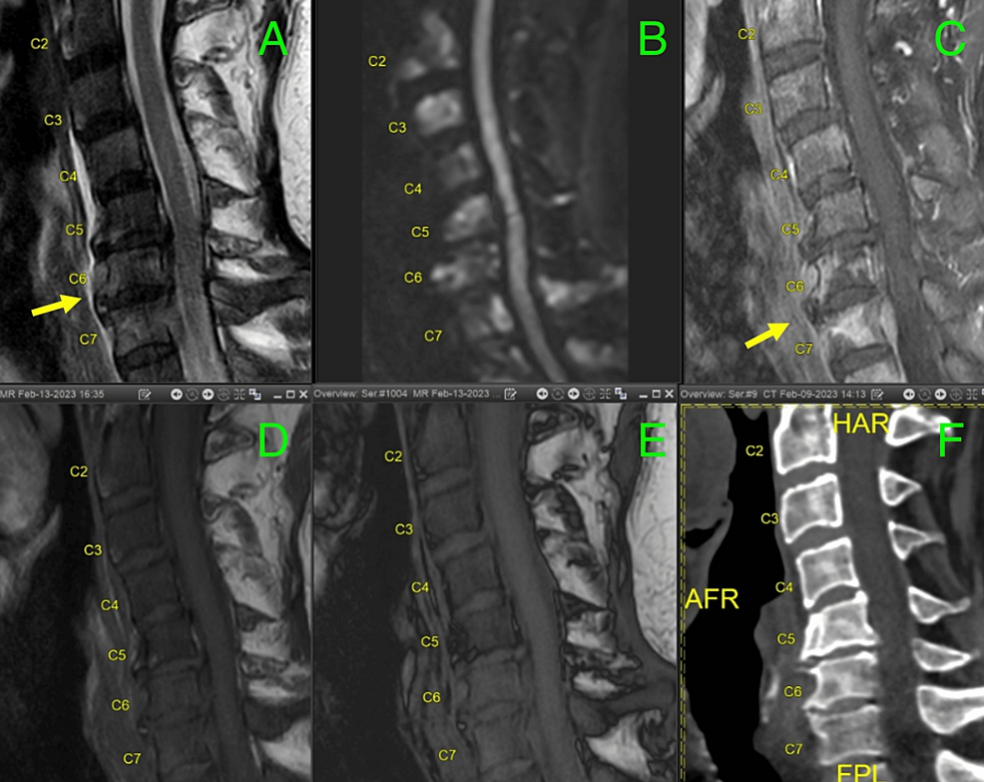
MRI cervical spine. (A) and (B) MRI cervical spine T1 and T2 with an abnormal marrow signal. (C) and (D) Diffusion-weighted signal imaging of (C) spine showing extensive signals from vertebral bodies suspicious of metastasis. (E) and (F) Enhancement on the vertebral body of C7 with enhancement behind it representing epidural spread and displacement of cord similar to seen on (A).
